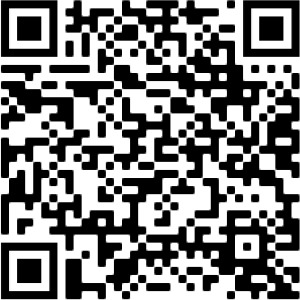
Preoperative computed tomography angiography of giant thoracoabdominal
